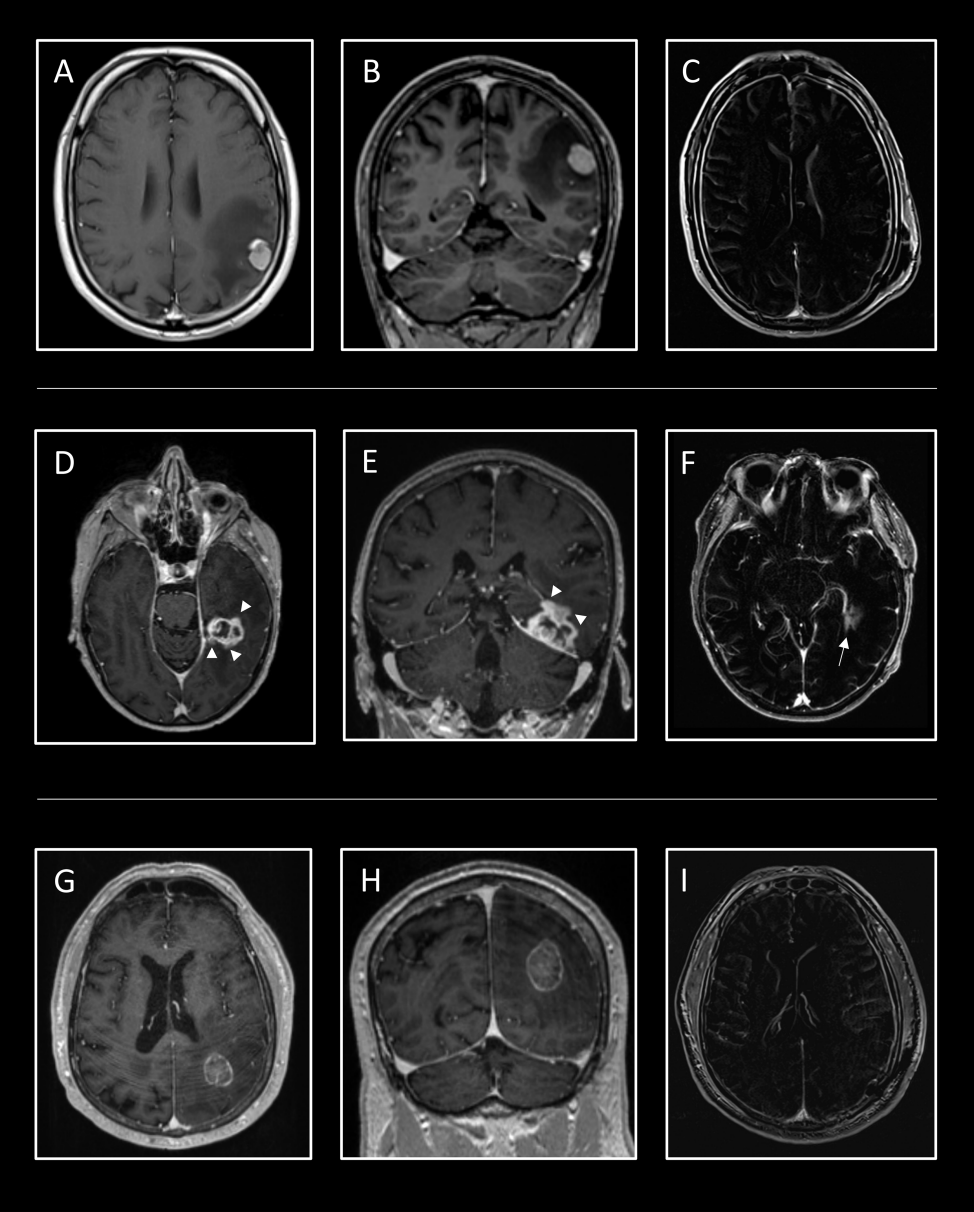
Case examples for the application of the regression tree. In the first line, the case of a 56-year-old man is shown who presented to the emergency department with an epileptic seizure and aphasia. Cerebral MRI showed a homogeneous contrast-enhancing cortical tumor in the parietal lobe with a volume of 2.2ml and extensive perifocal edema (A, B). The risk of uSTR is very low at 4.8%, thus a GTR was confirmed in the postoperative T1 subtraction sequence (C). Additionally, the preoperative aphasia improved. The second case shows a 69-year-old woman whose temporal metastasis of esophageal carcinoma grew progressively to 9.5ml despite radiation (D, E). Neurological examination revealed mild aphasia. Detailed analysis revealed an irregular/diffuse contrast agent enhancement (white arrowheads), which, in combination with a distance of 15.4mm from the cortex, indicates a high risk of an uSTR at 43.2%. The aphasia improved postoperatively, but a residual tumor (white arrow) of 0.6ml (EOR_rel_ = 94%) was detected (F). At the bottom, the MRI of a 70-year-old man who suffered from ataxia and visual disturbance presents a subcortical tumor in the parietal lobe with a volume of 9.0ml (G, H). The tumor has circumscribed contrast agent enhancement, with 11mm distant from the cortex, thus the risk for uSTR is moderate at 15.4%. Postoperatively, the ataxia and visual impairment improved and a GTR was obtained (I).
